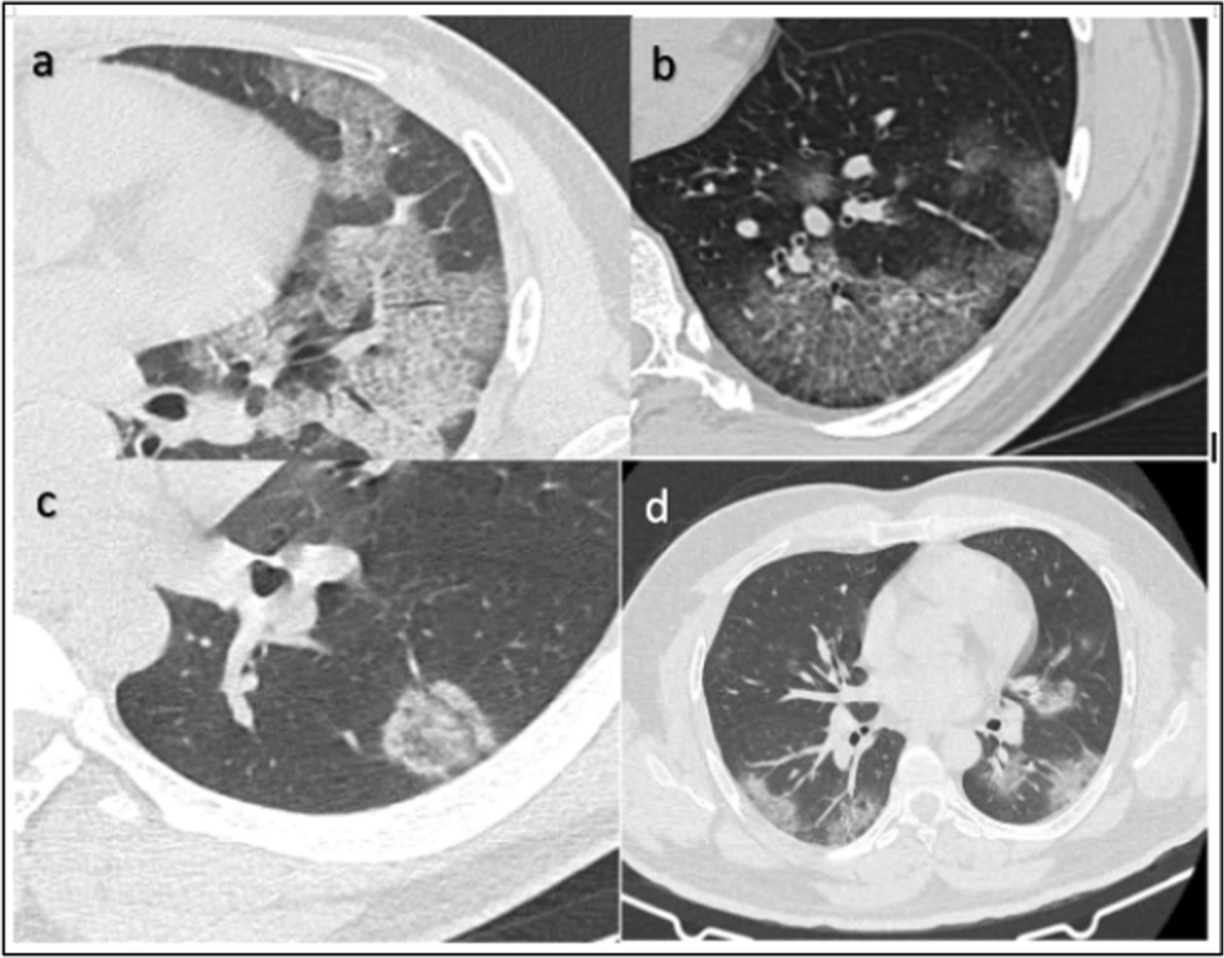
Axial HRCT images of different patients showing typical imaging features in COVID-19—crazy paving pattern (a, b), reverse halo sign (c) and peripherally arranged ground-glass opacities (d)
